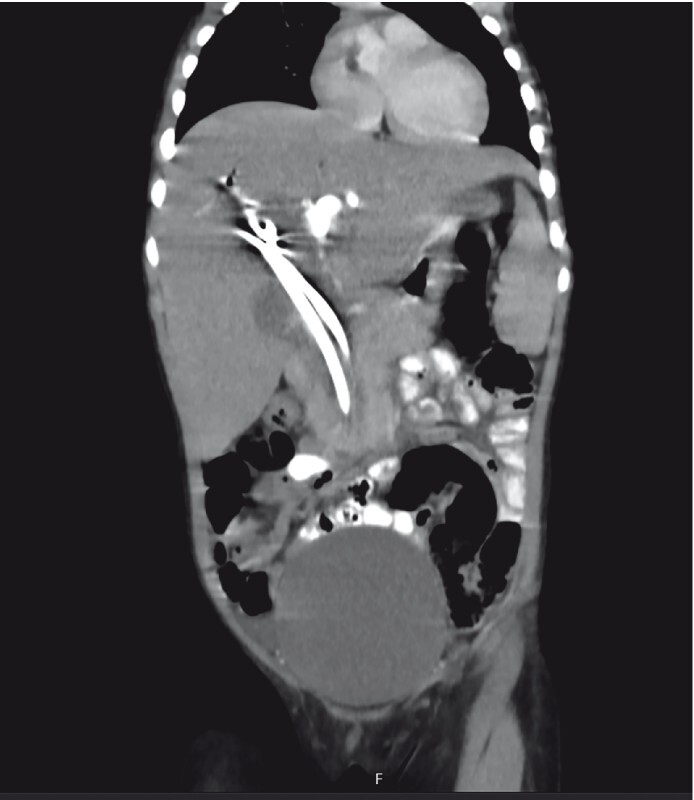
Abdominal computed tomography showed dilation of the intra- and extrahepatic bile ducts, an increase in the length of the gallbladder, and an increase in the density of the common bile duct in the distal part of the gallbladder, in a heterogeneous and occasionally tubular appearance. A parasite was considered.
